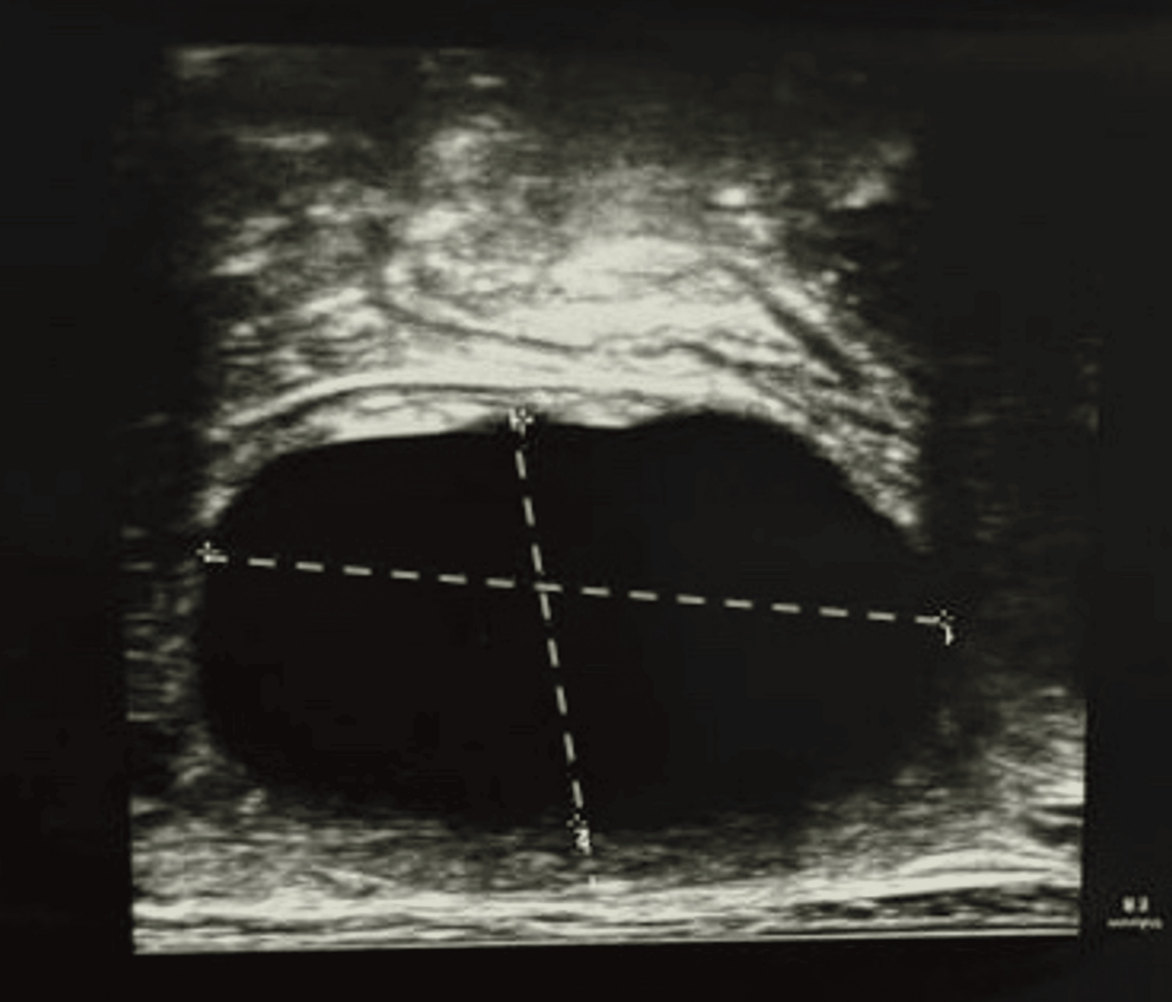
Ultrasound findings of the abdominal lesion. Ultrasound of the abdominal lesion showing a purely cystic lesion with no solid components or membranous structures, consistent with a stage I hydatid cyst of Gharbi classification.
